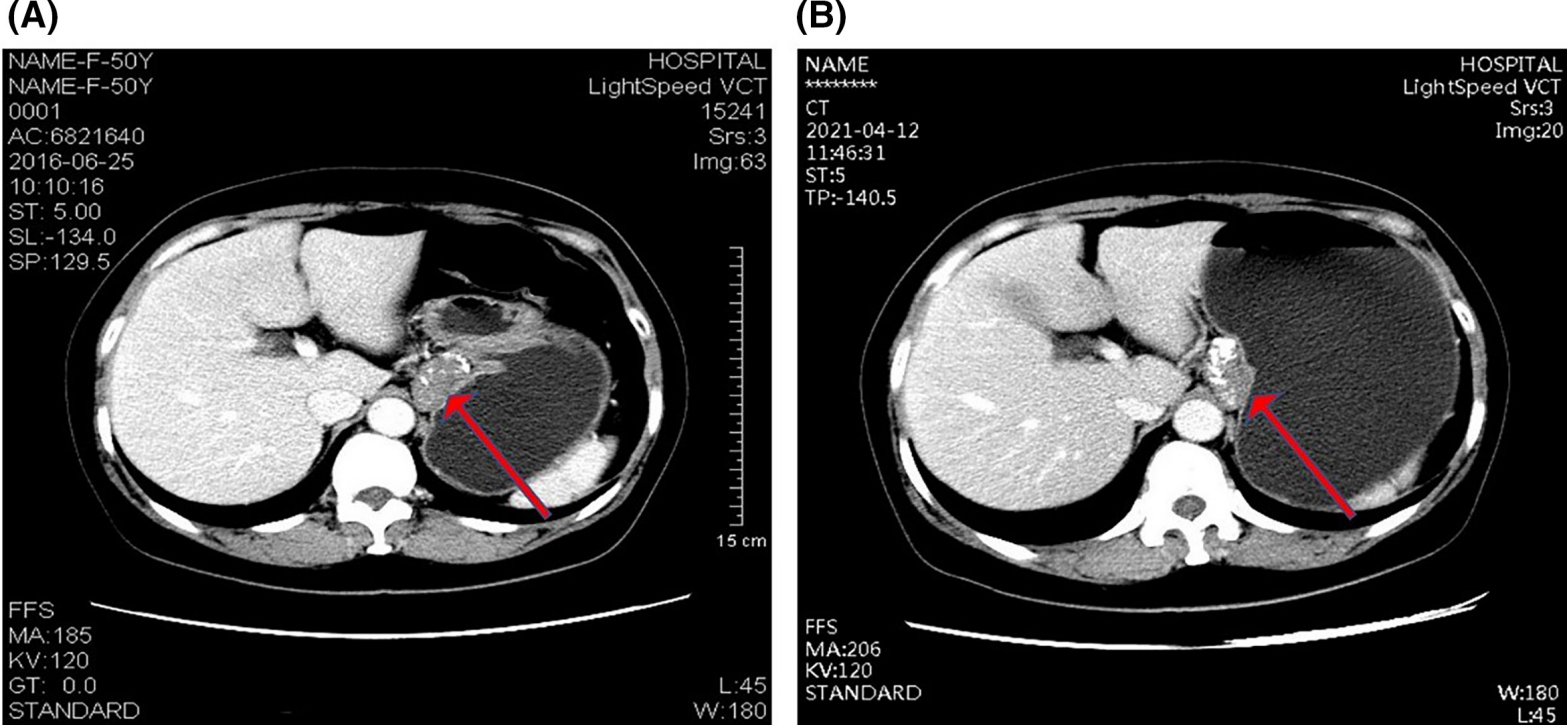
Conventional and enhanced computed tomography (CT) images. A submucosal mass protruding into the abdominal cavity was seen in esophagogastric junction. (A) the CT image 5 years ago; (B) the CT image on admission.
